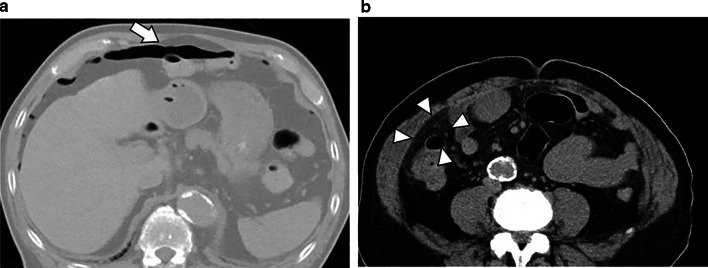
Computed tomography revealed intraperitoneal free air (a: arrow) and dirty fat signs around the ascending colon (b: arrowhead)
